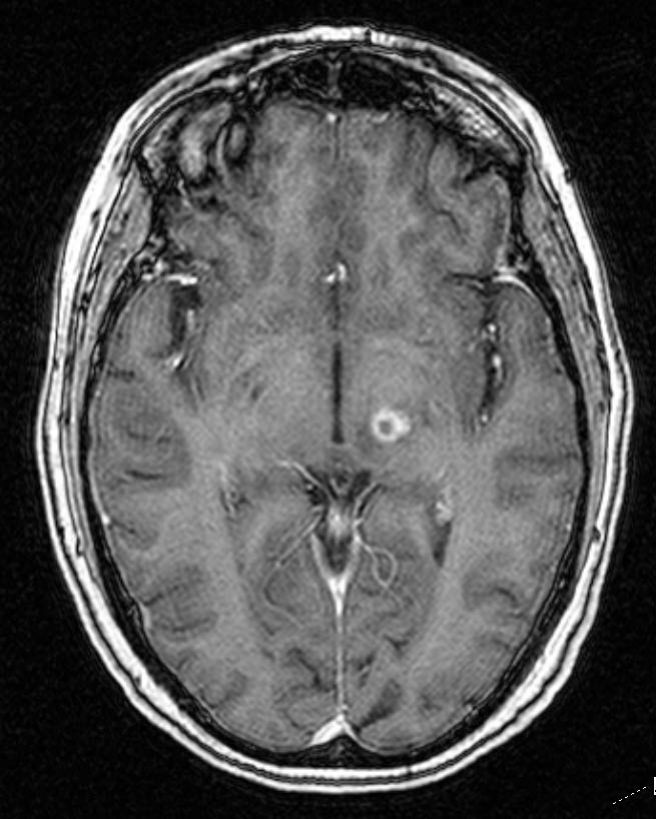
MRI 8 months after radiosurgery.
